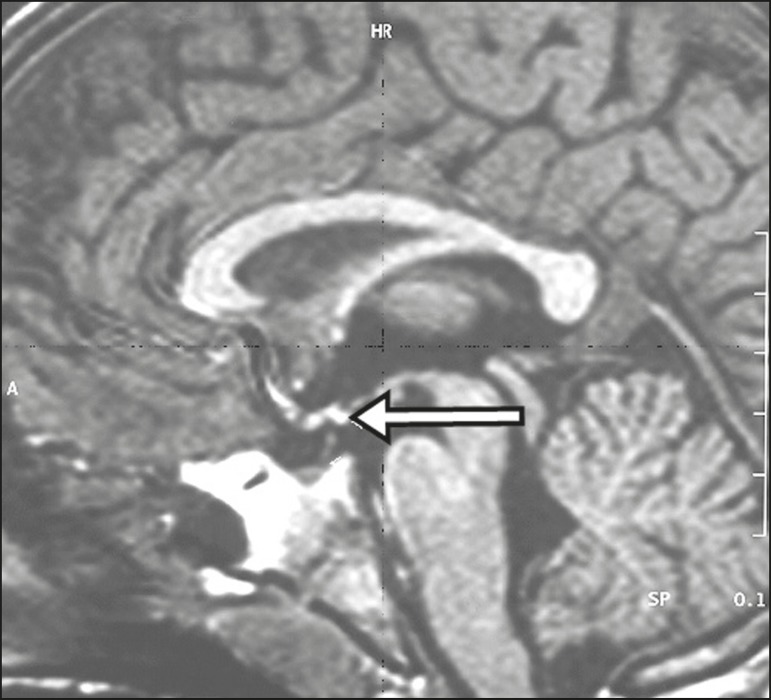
Sagittal T1-weighted MRI sequence showing an ectopic neurohypophysis
